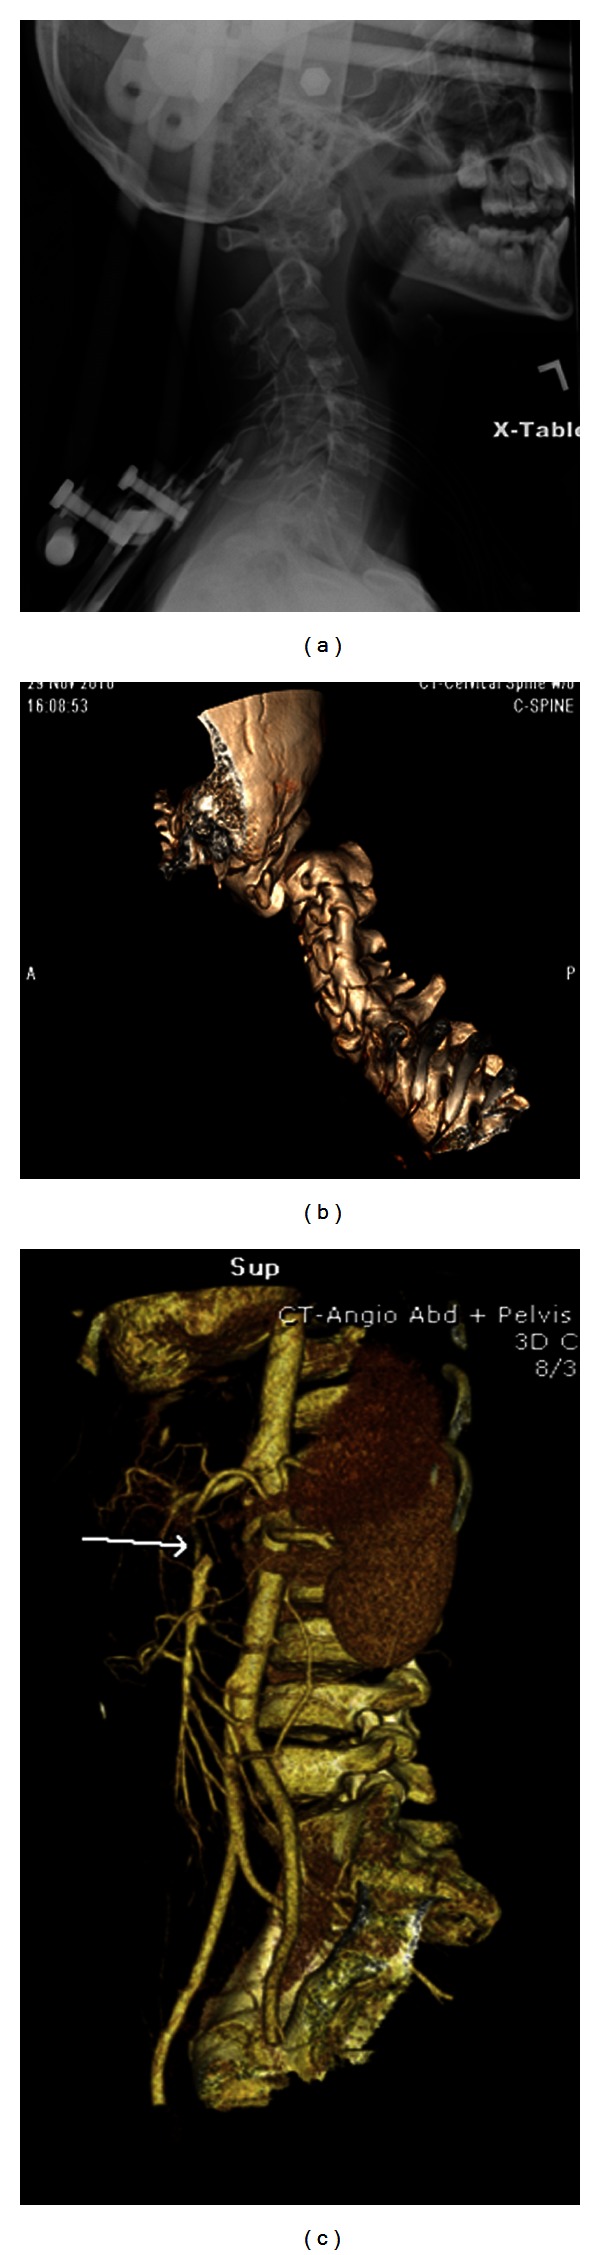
Lateral view (a) and 3D CT reconstruction (b) of the cervical spine of the patient. Note the severe posterior C1/C2 subluxation with posterior displacement of the C2 vertebral body in relation to C1. (c) 3D reconstruction from a CT angiogram for the patient, showing the filling defect of the inferior mesenteric artery (arrow).
